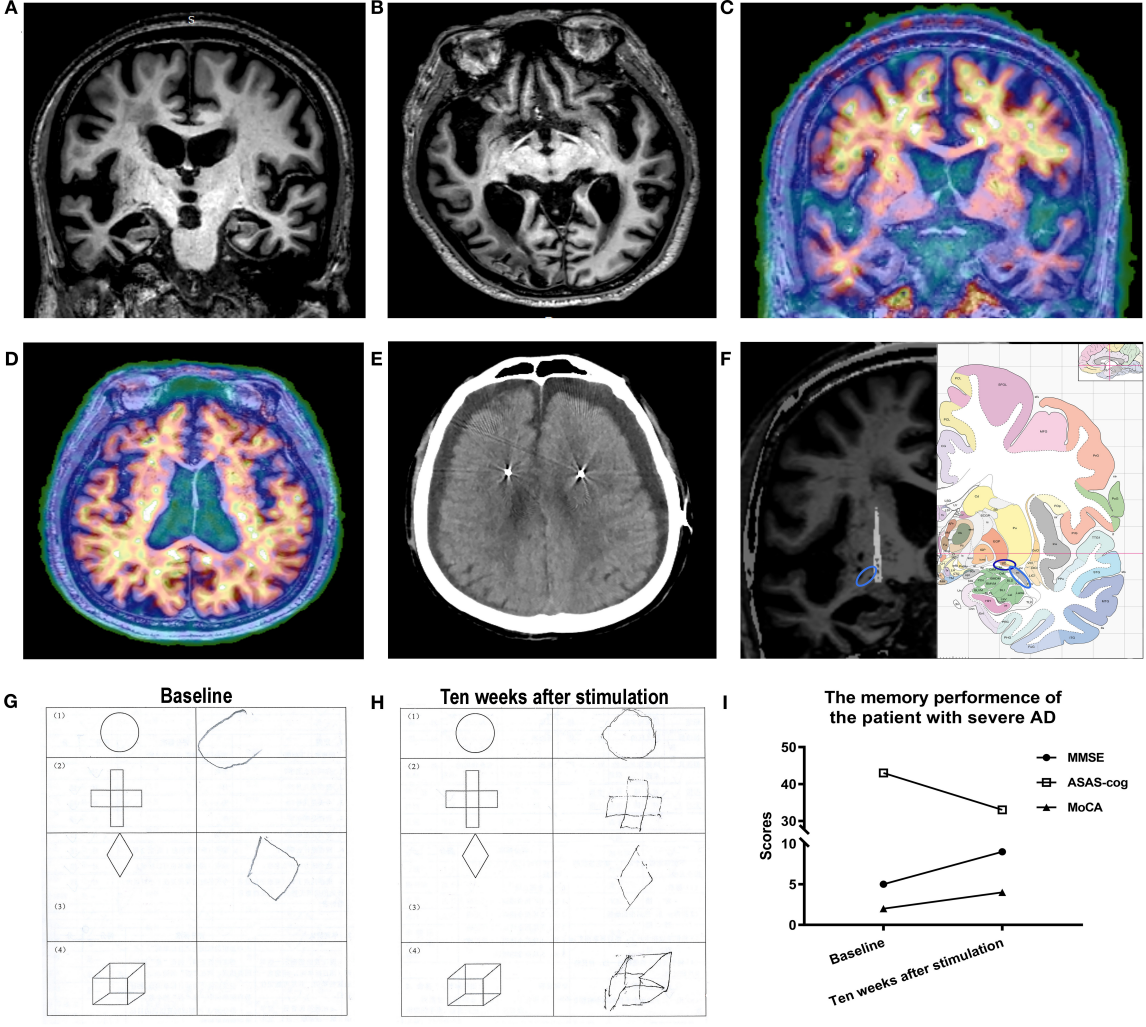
(A,B) A preoperative MRI revealed global atrophy, especially in the hippocampi and temporal lobes. (C,D) PET imaging showed β amyloid widely deposited in the brain. (E) Bilateral subdural effusion was identified by CT 1 week after surgery. (F) A fused image (left) of the patient's preoperative MRI and postoperative CT showed that the lead (arrow) was accurately implanted into the nucleus basalis of Meynert (NBM) (dark blue circle = NBM; light blue circle = anterior commissure). Atlas (right) of the human brain. (G,H) Patient performance before and after NBM-deep brain stimulation (DBS). The patient was able to draw all geometric shapes after NBM-DBS but performed poorly before NBM-DBS. (I) Changes in memory performance by the patient over time.
